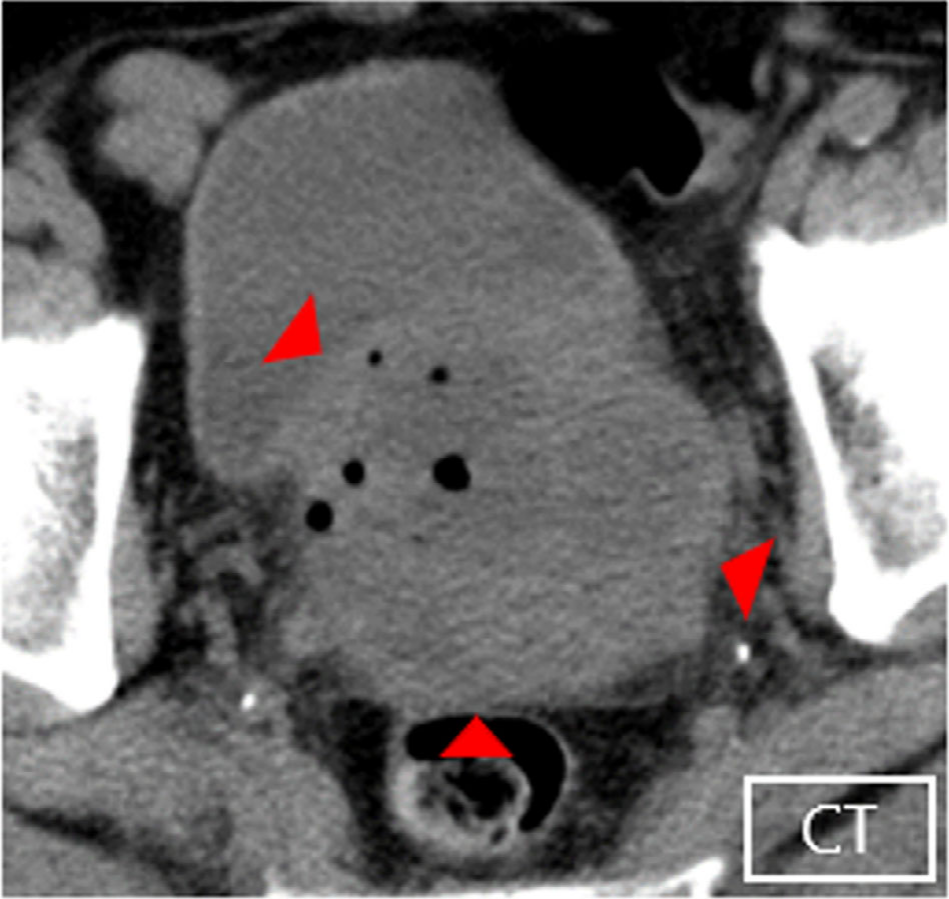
CT revealed a large multilocular mass with small amount of air.
